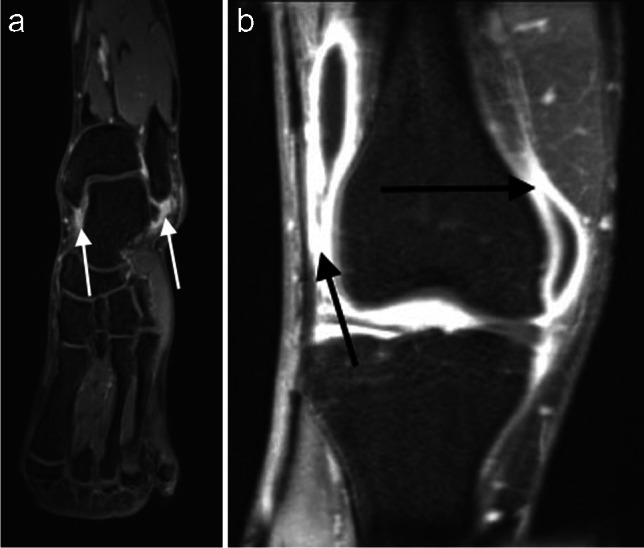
a Water-sensitive T1-weighted VIBE Dixon post-gadolinium image of the ankle in a patient with rheumatoid arthritis with synovitis of the tibio talar joint (white arrows). b Coronal water-sensitive VIBE Dixon post-gadolinium image of the knee in the same patient with marked synovitis seen within the joint (black arrows) and a moderate sized joint effusion
